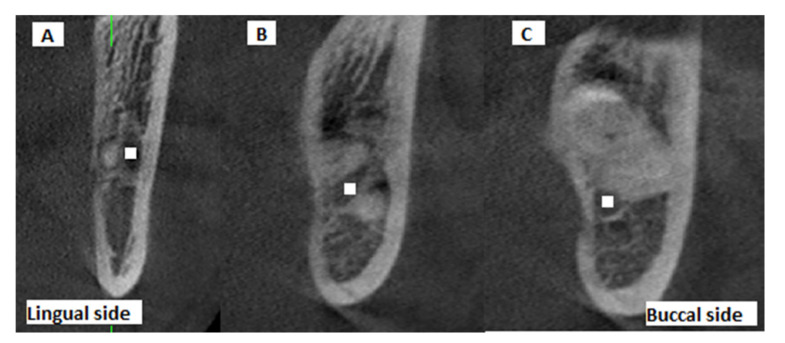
Cross-sectional CBCT views showing the buccolingual position of the IMTM apex relative to the MC: (A) buccal, (B) inter-radicular, (C) lingual (white squares: center of mandibular canal).
